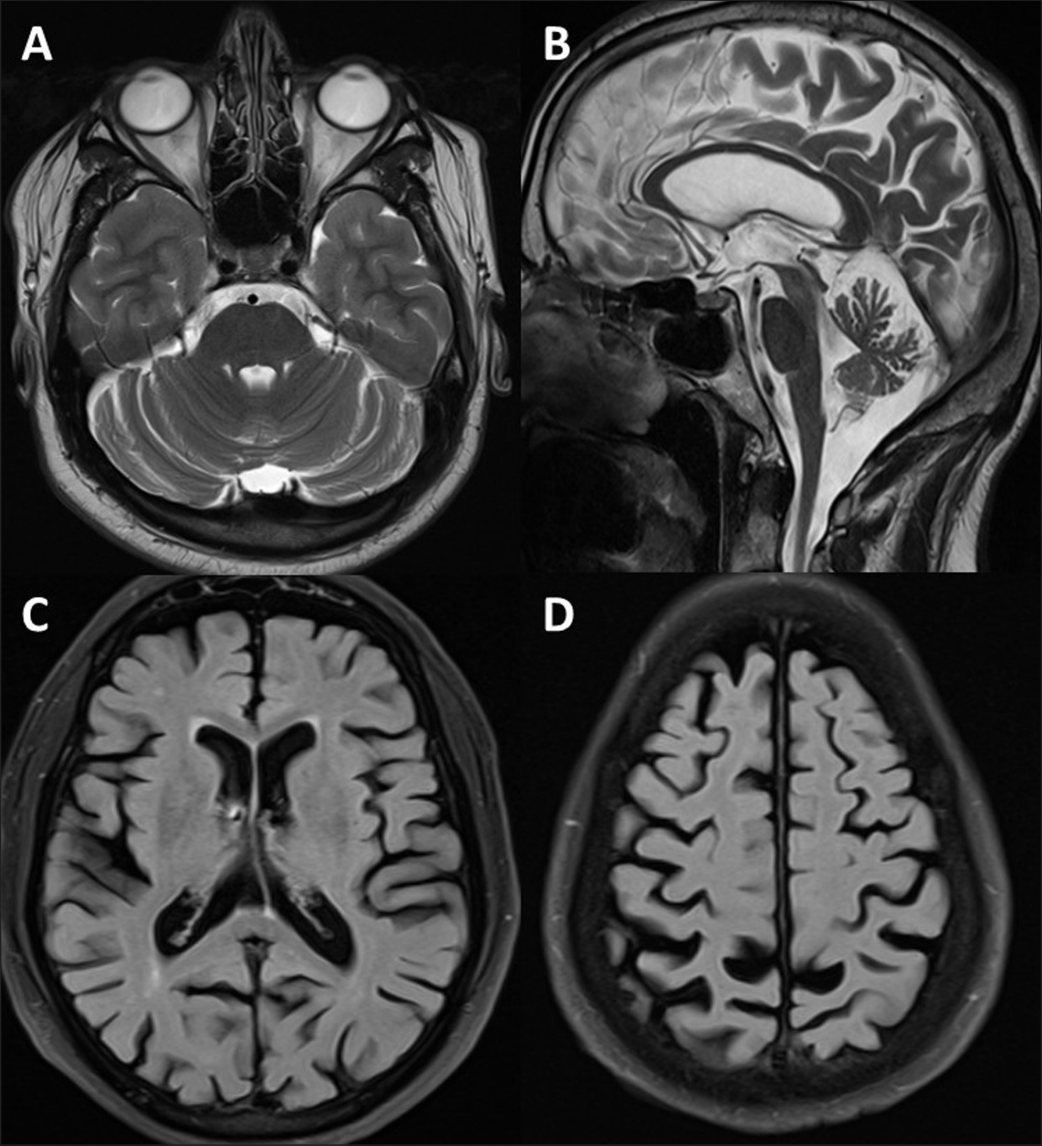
MRI brain showing cerebellar atrophy in (A) T2 axial and (B) mid-sagittal sections and cerebral atrophy in (C, D) FLAIR axial sections.
